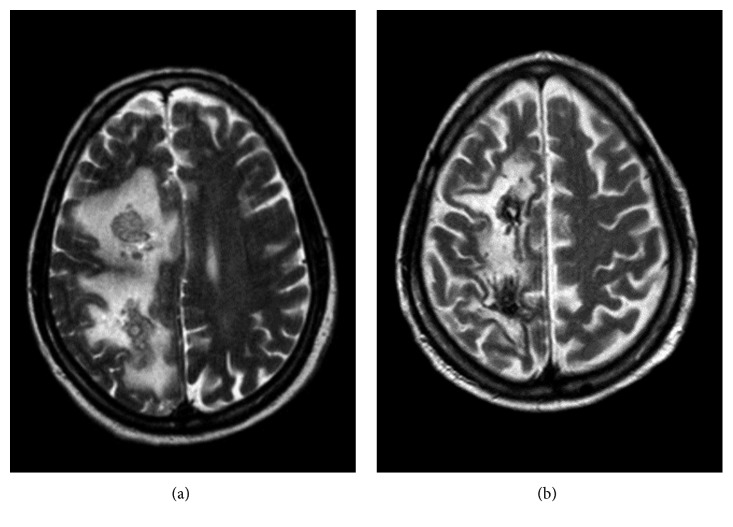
MR image showing a caudal extension of the lesion with irregular enhancement with irregular enhancement and a necrotic region (FLAIR/long TR).
